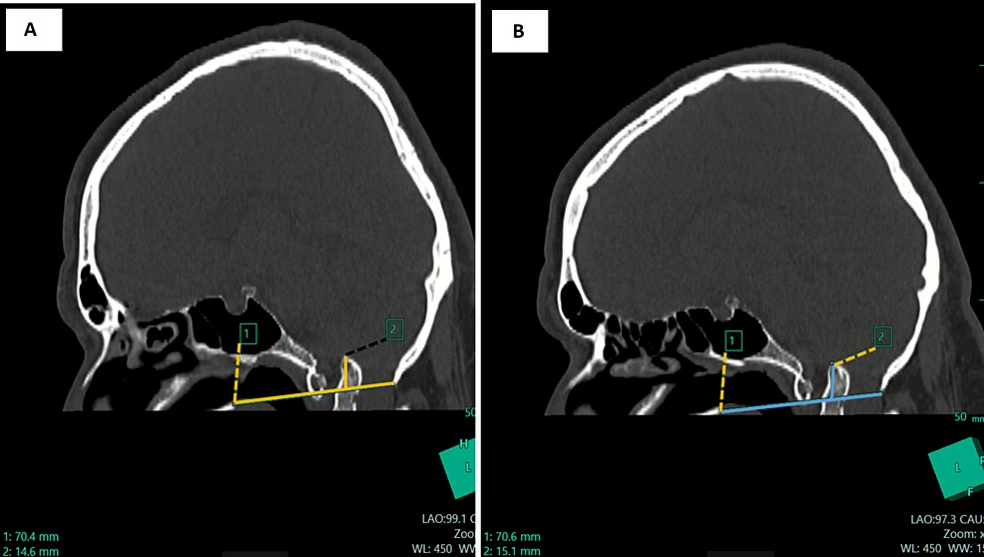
Sagittal CT images show that the Chamberlain line, tip of the odontoid process, is ~14.6 mm above this line (A) and the McGregor’s line, tip of the odontoid process is ~14.8 mm above this line (B)
